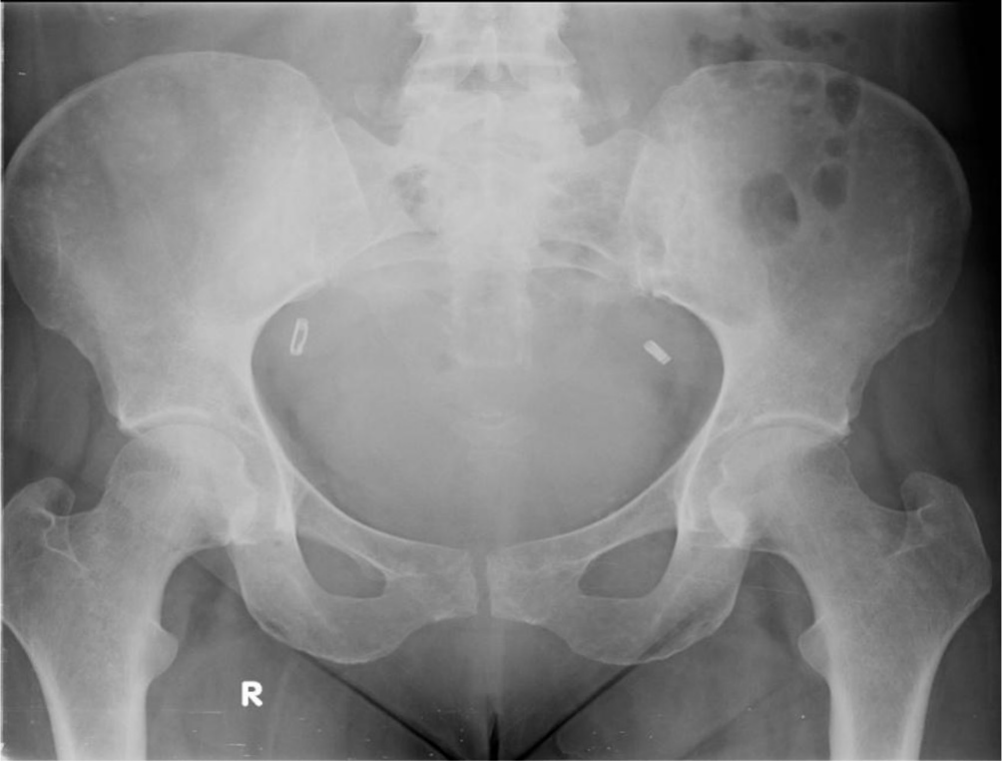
X-ray of pelvis of a 50-year-old female with node-positive breast cancer (Case 2) showing multiple sclerotic lesions in the iliac wings and proximal femora.
